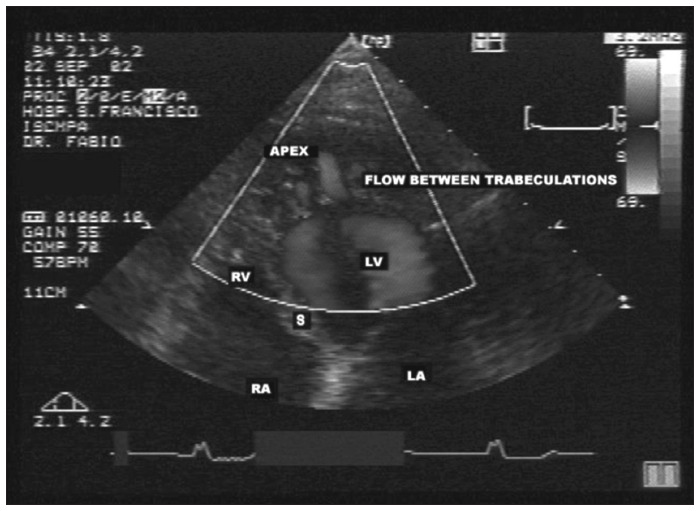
Apical four-chamber view using color Doppler, demonstrating the flow between the prominent trabeculae. This image was obtained from the same patient as in Figures 1 and 2.
